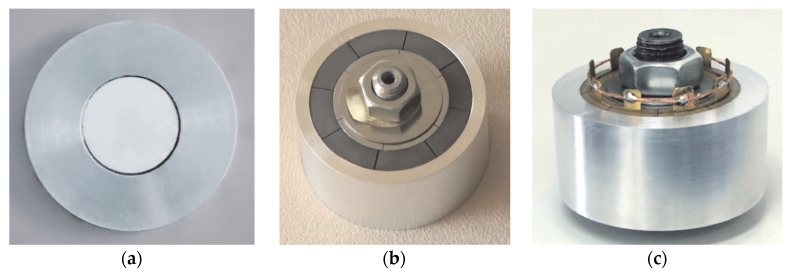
Three radial piezoelectric ultrasonic transducers. (a) Radial discs. (b) Radial cylinders. (c) Sandwiched rings.
